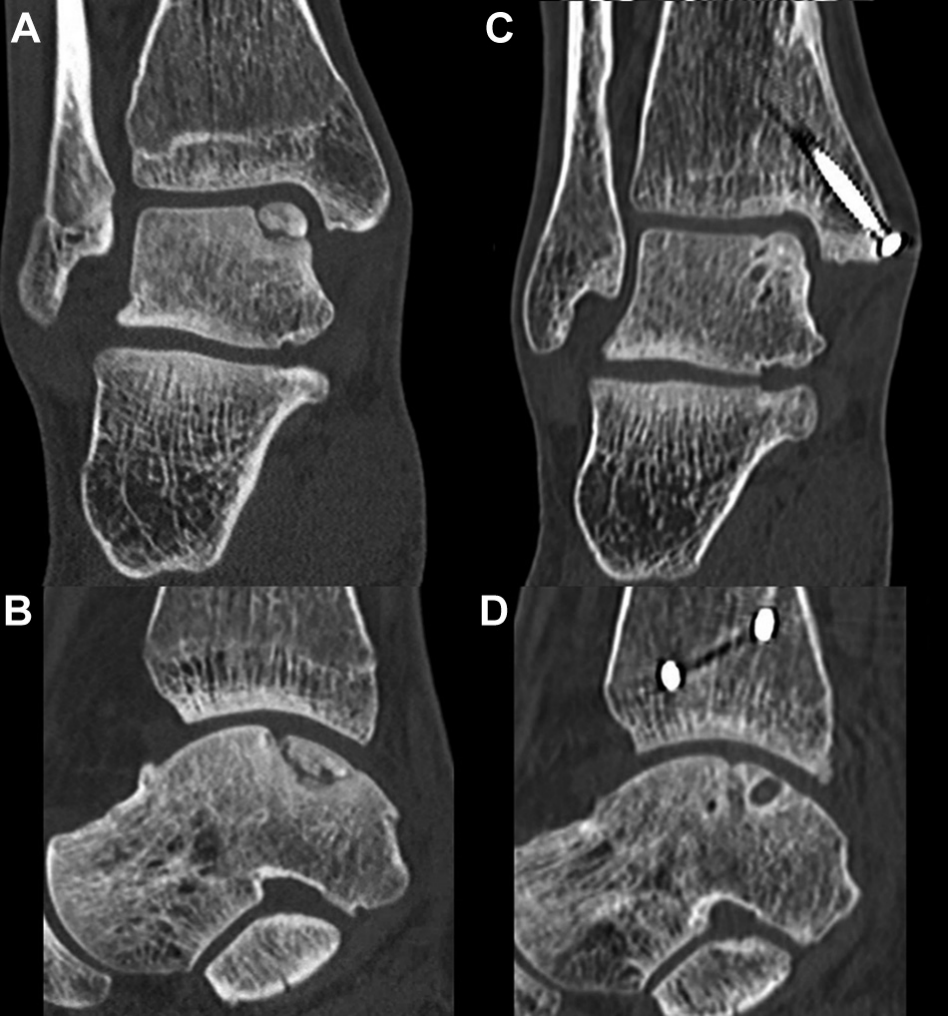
Preoperative coronal (A) and sagittal (B) and 2-year postoperative (C, D) computed tomography images of an 18-year-old male with a posteromedial osteochondral fragment of the talus, who underwent open lift, drill, fill, and fix with 1 bioscrew. The postoperative radiolucency on the talus is the bioscrew in situ.
